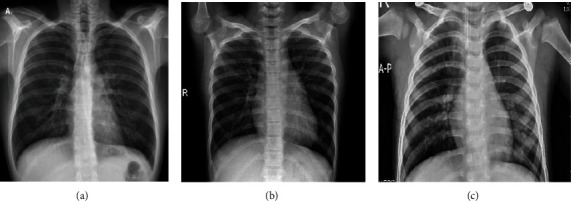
Sample images of the (a) COVID-19, (b) normal, and (c) pneumonia chest category X-rays.
